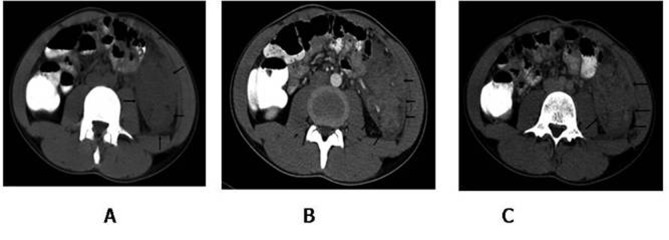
Figure 1: Axial computed tomography image show a soft tissue mass, located between left psoas muscle and lateral abdominal wall. The lesion partially surrounds the sigmoid colon (A). Contrast enhanced CT arterial (B) and venous (C) phase show marked heterogeneous involvement.
